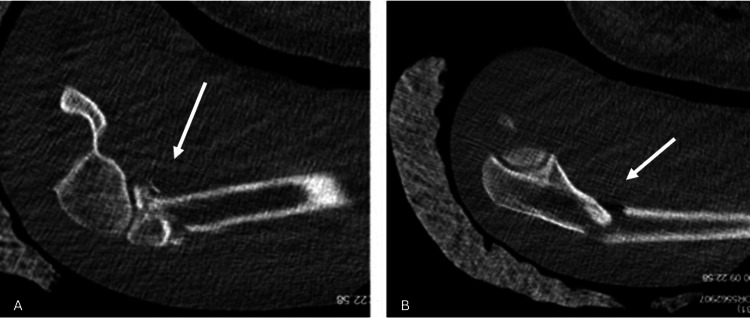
Sagittal section of the computed tomography images of the patient. Radial head fracture with subluxation (A) and proximal ulna fracture (B) can be seen in the tomography sections White arrows indicate the fracture at the proximal ulna.
